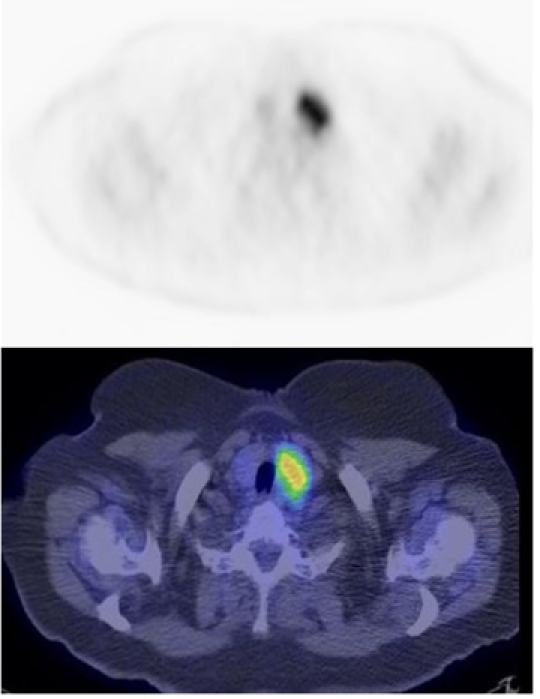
FDG PET and fused PET CT images (axial view) at the level of the thyroid gland. Focal intense uptake of tracer was incidentally found in the left lobe of the thyroid [Image courtesy of Centre for Molecular Imaging, Peter MacCallum Cancer Centre, East Melbourne, Victoria, Australia].
